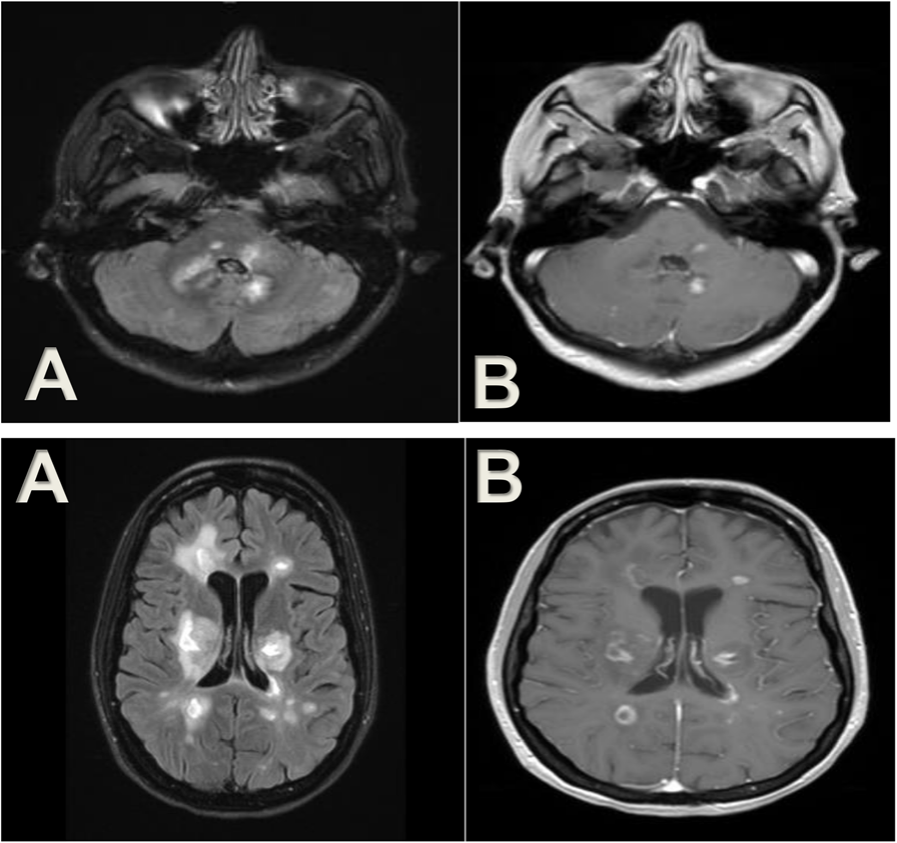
MRI of brain: a. Axial FLAIR b. Axial Post-Gadolinium. Patient had a severe MS relapse after fingolimod discontinuation for 12 weeks and on treatment with DMF for 4 days. MRI of brain showed multiple enhancing lesions with a nodular and ring enhancement pattern in the brainstem, posterior fossa, and cerebral hemispheres bilaterally (Case 2)
